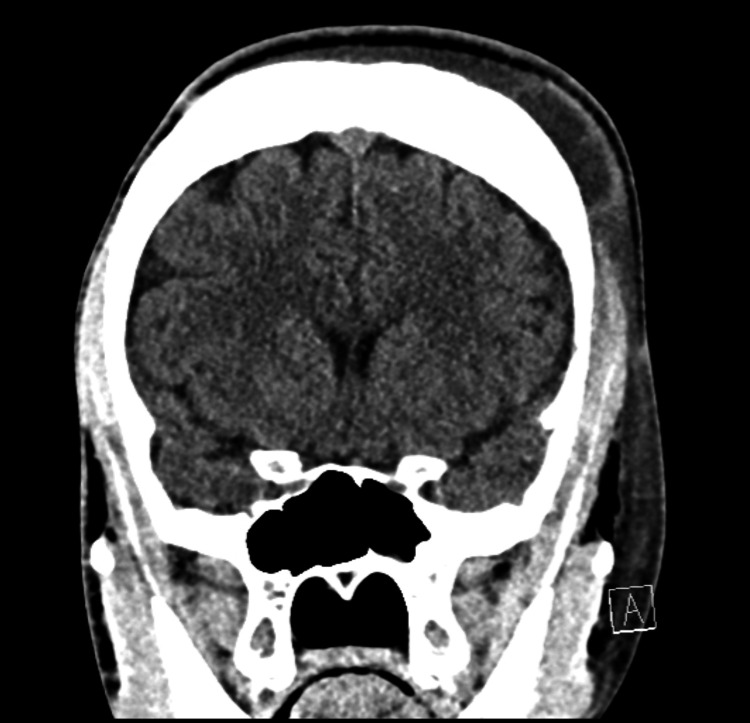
Soft tissue swelling noted on head and maxillofacial CT
